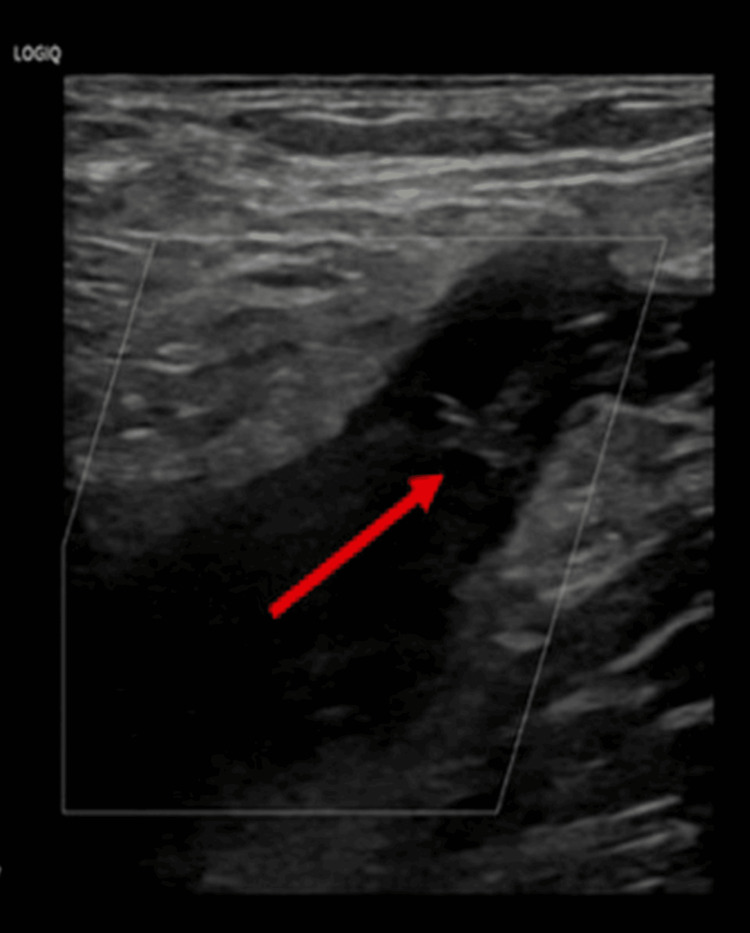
Doppler ultrasound of the right CFV showing intraluminal echogenic thrombus (arrow) with loss of compressibility, consistent with acute deep vein thrombosis involving the right external iliac, common femoral, and femoral veins CFV: common femoral vein
